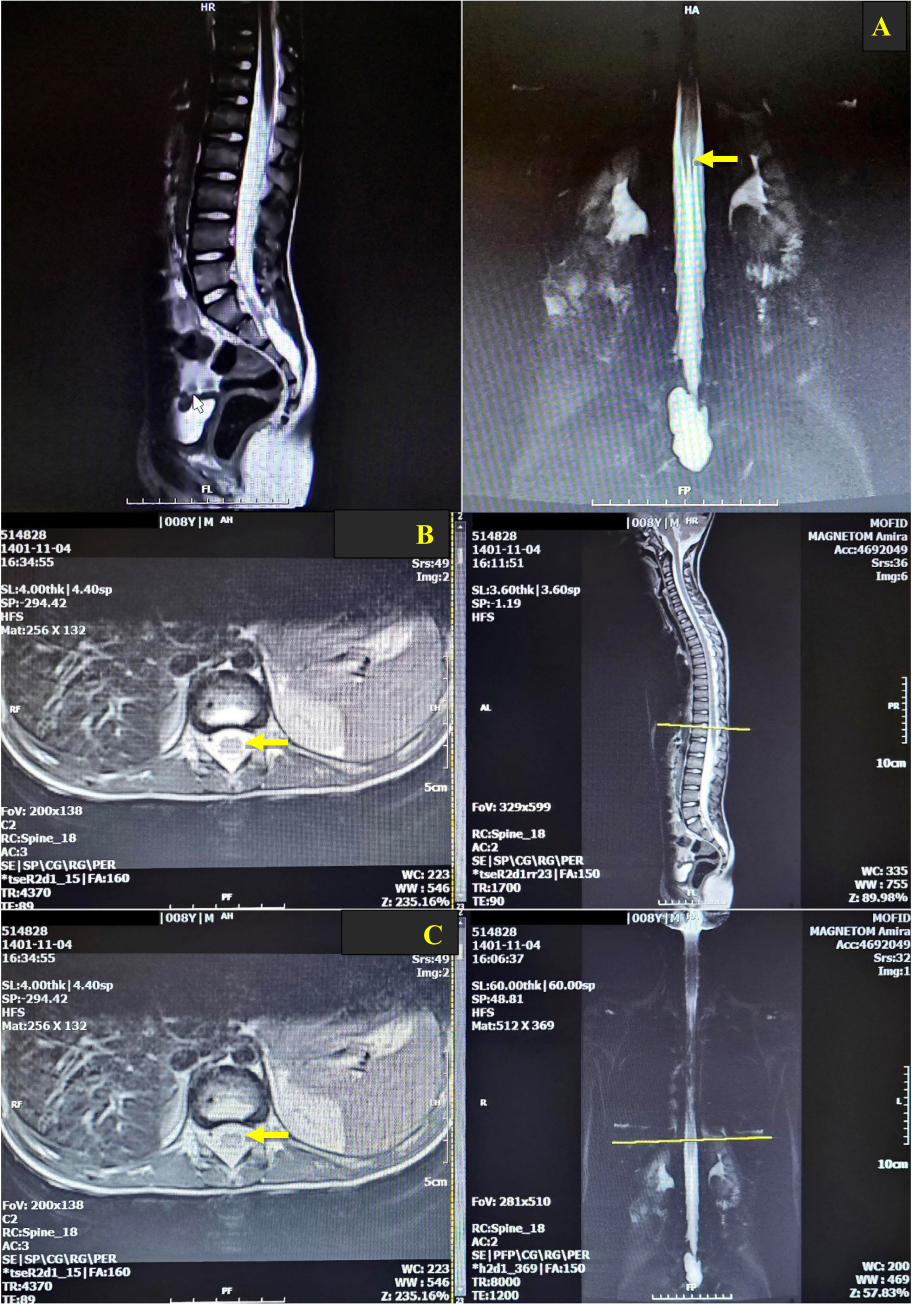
MRI of spine with increased enhancement in the cauda equina region, particularly in the ventral roots (A–C).
